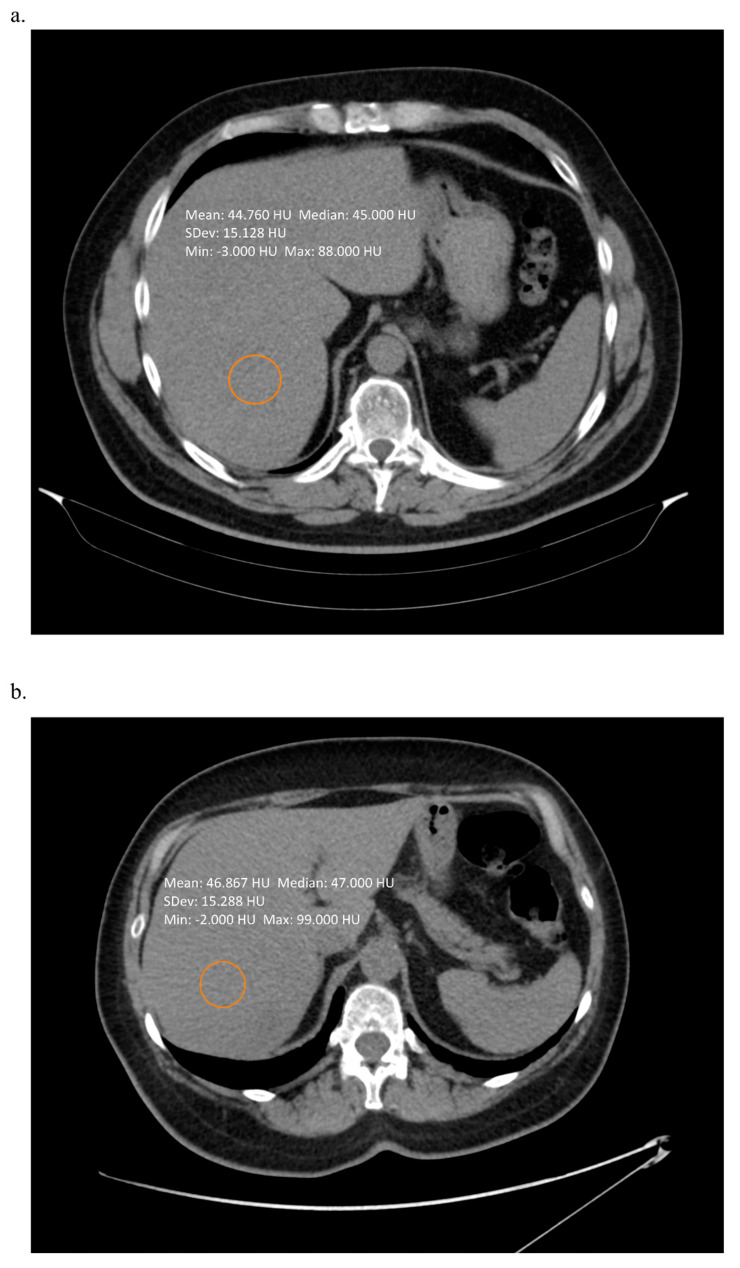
Non-contrast enhanced computed tomography scan of a patient with (a) 60% of hepatic steatosis (b) 80% of hepatic steatosis. In both cases simple measurement of the liver density was not suggestive on such severe changes. Images from the authors’ department.
